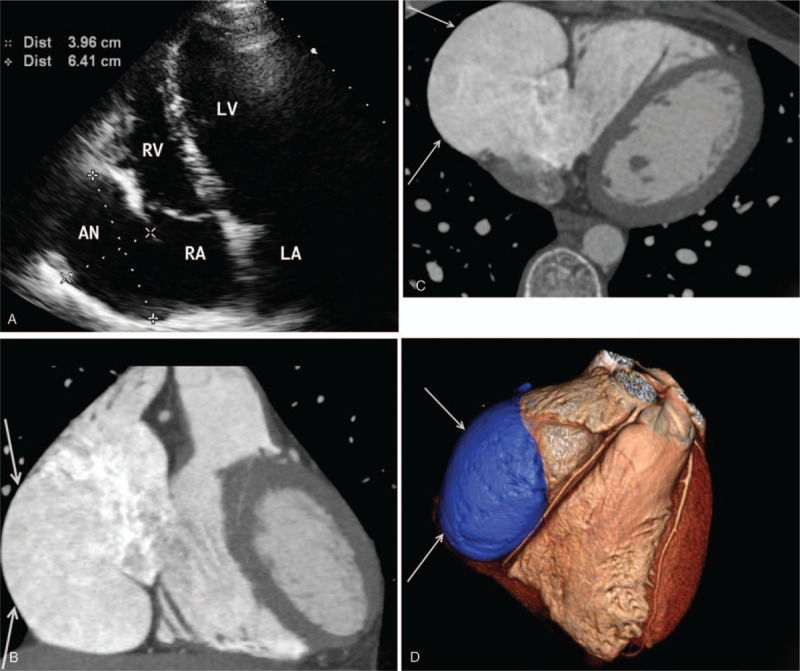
Ultrasonography and enhanced CT images obtained in case 2. (a) An ultrasound image revealed a cystic mass that connected the right edge of the heart with the right atrium. (b and c) Coronal and axial enhanced CT images revealed an oval-shaped diverticular lesion in the right atrium that had a thin wall and was connected to the right atrium. (d) A 3D image revealed no abnormality in the adjacent coronary artery.
